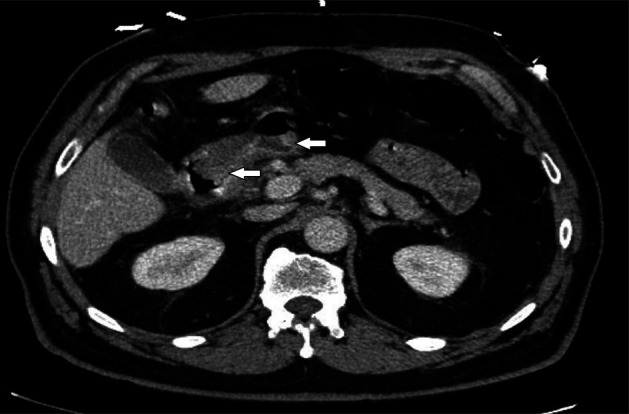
Abdominal CT scan showing inflammatory changes of the gastric antrum and proximal duodenum.
